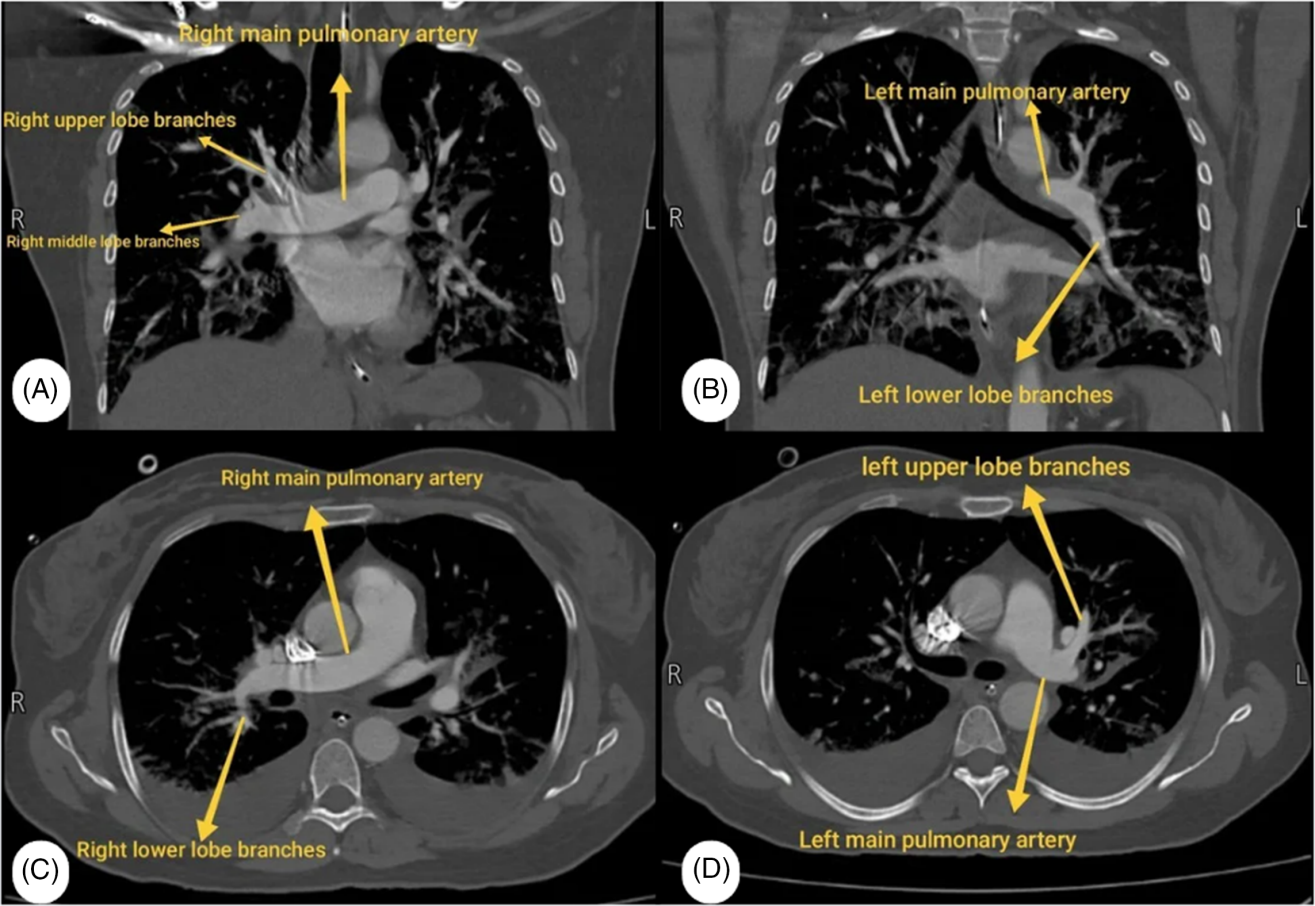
(A, B) Coronal chest CT (mediastinal window) showing the pulmonary arteries. (C, D) Axial view.
